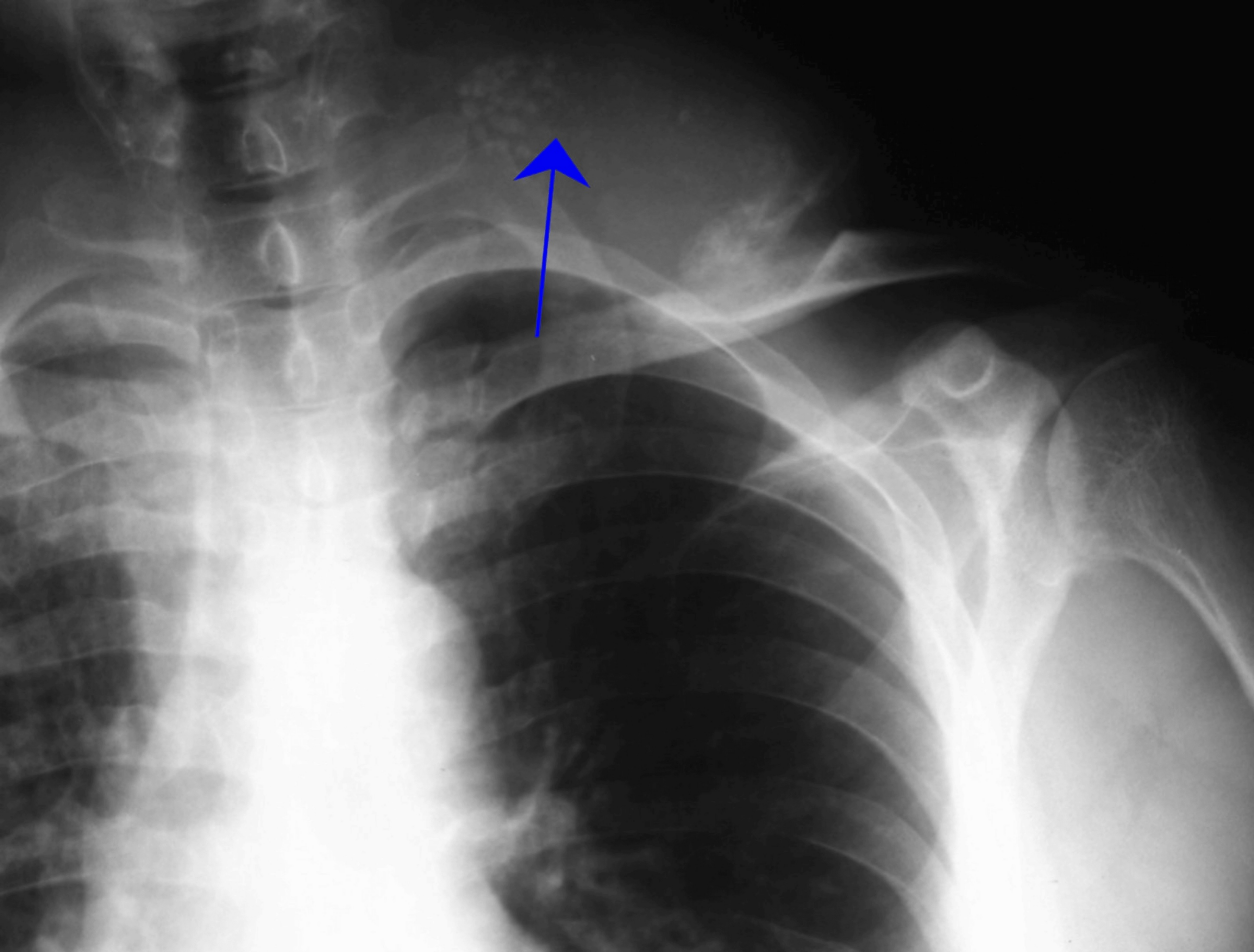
X-ray of the left clavicle shows a lesion on the medial and superior aspect of the clavicle
